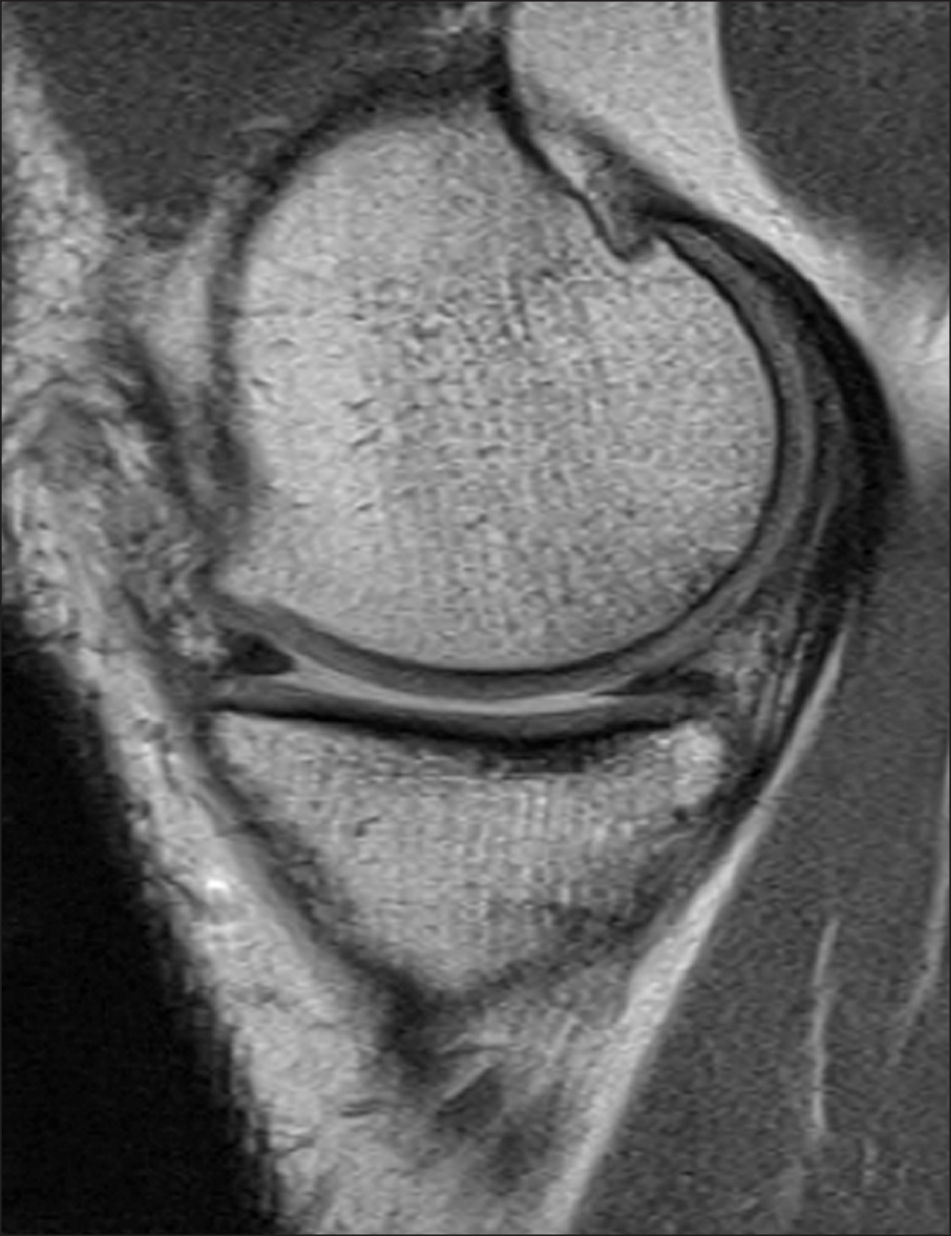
Sagittal T1-weighted fast spin echo MR-image demonstrating a small but intact collagen meniscus implant at the posterior horn of the medial meniscus.
